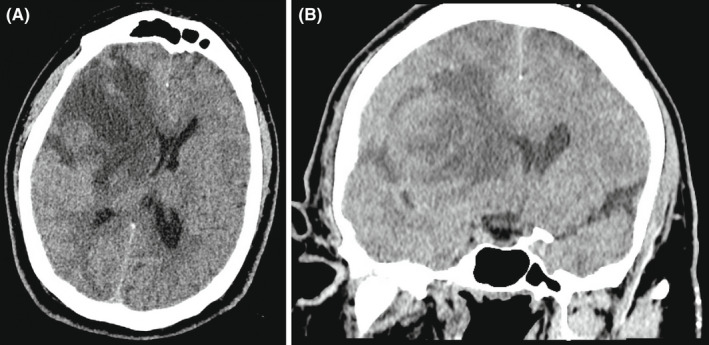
Axial (A) and coronal (B) head computed tomography (CT) without contrast demonstrates a right frontal lesion causing mass effect and midline shift
